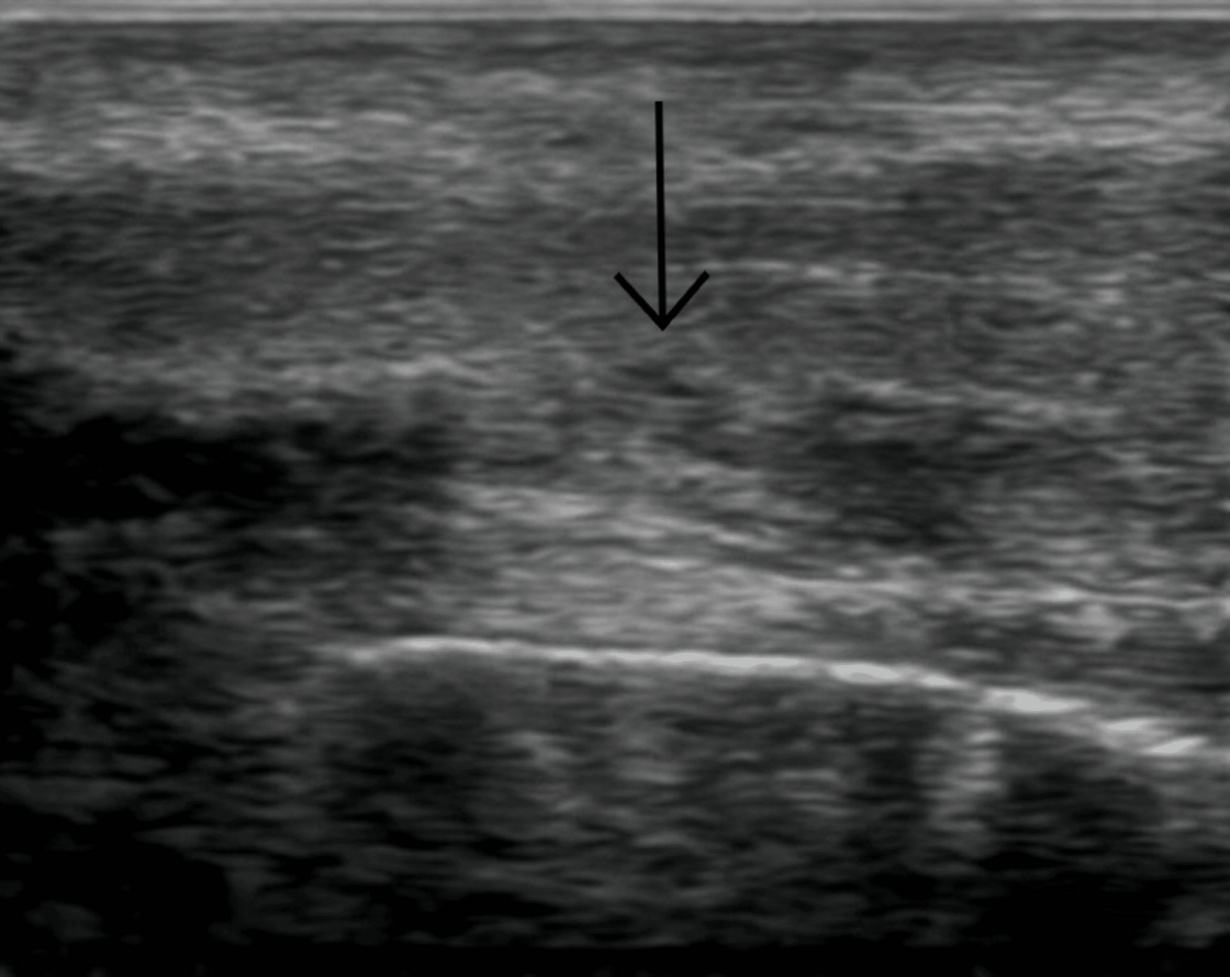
Ultrasound image of the penis revealing a 1 cm discontinuity in the tunica albuginea. The black arrow indicates the site of the tunica albuginea fracture.
